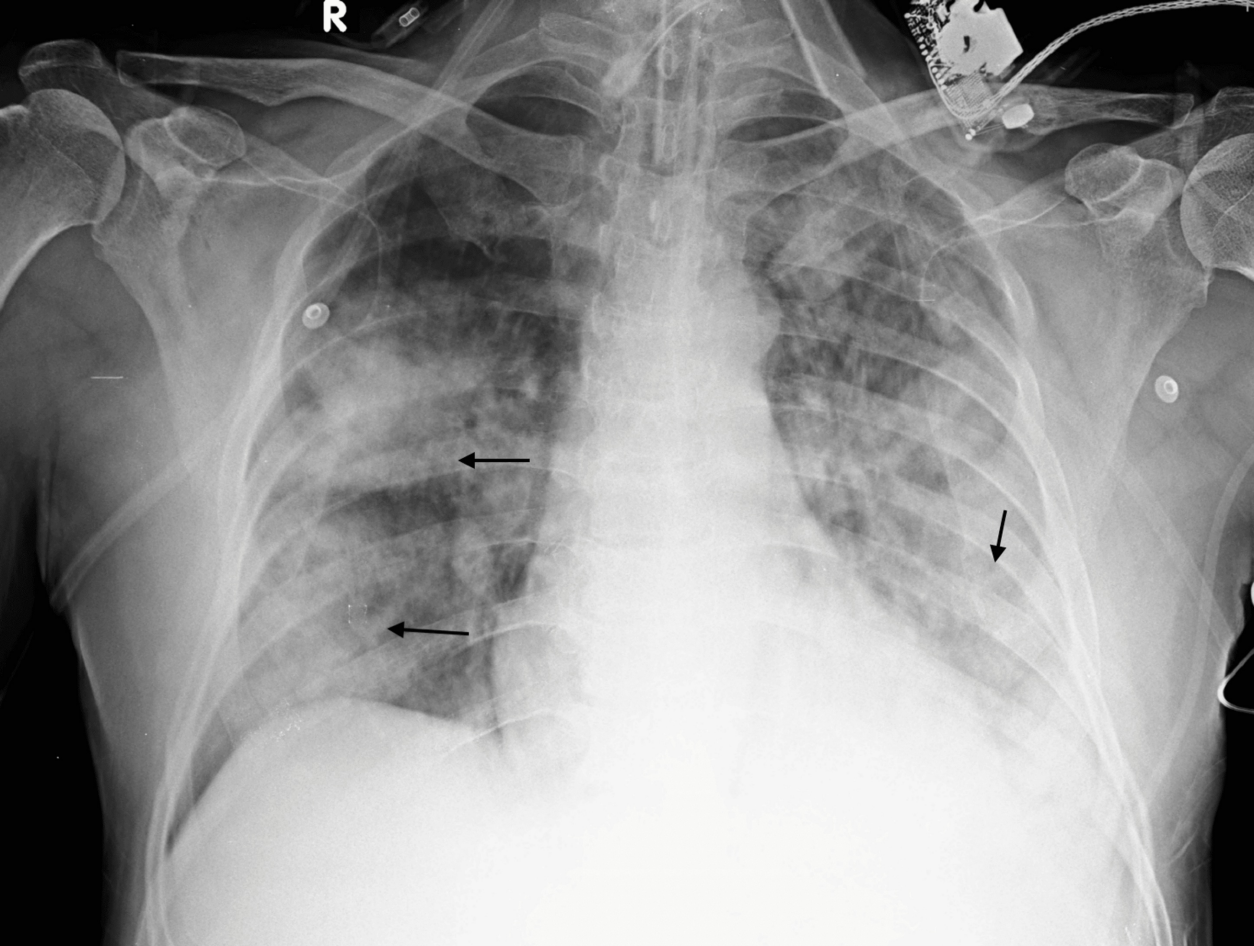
Chest X-ray on presentation showing consolidations with air bronchograms in bilateral mid and lower zones of the lungs, along with fluffy non-homogeneous opacities consistent with pneumonia and early ARDS ARDS: Acute respiratory distress syndrome
